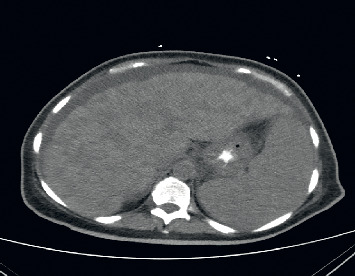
Computed tomography (CT) abdomen showing hepatosplenomegaly, numerous hypodensities in the liver.
